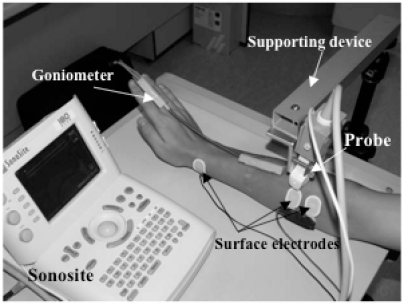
The experimental setup for collecting the ultrasound images, SEMG, and wrist angle.
